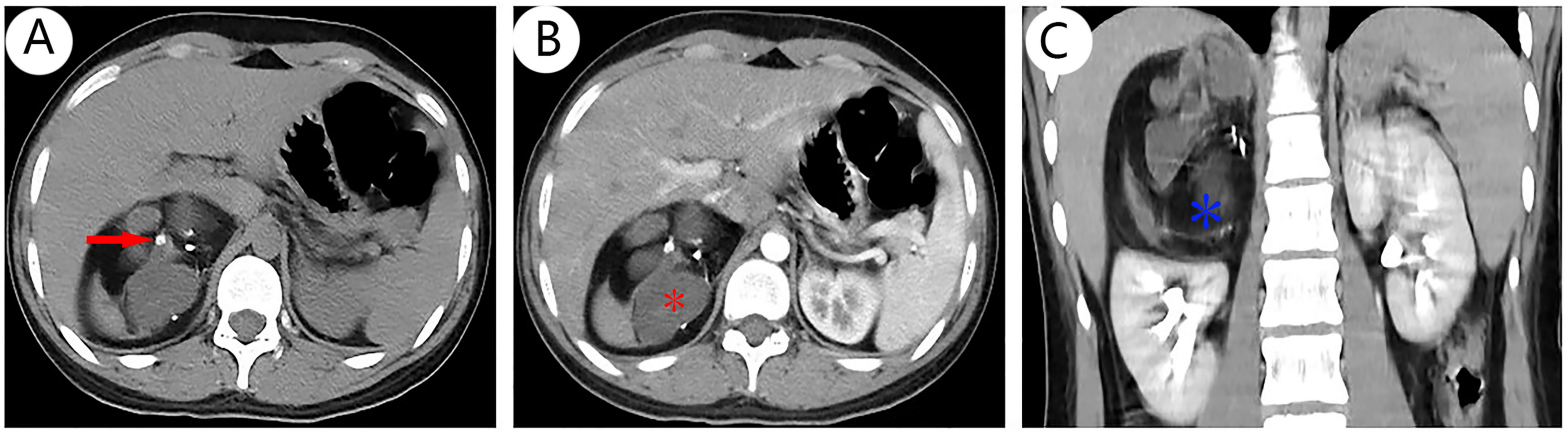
CT scan + contrast-enhanced ultrasound + 3D of adrenal gland revealed a spherical mass arising from the right adrenal gland with mixed density that was suspected to malignant tumor, a solid with multiple high-density nodules [(A), red arrow], some cystic-like lesions [(B), red *], and fat density shadows [(C), blue *], and no obvious enhancement signals (B, C). Other abdominal structures were normal and there was no evidence of distant metastasis although the right kidney was slightly displaced by the mass.
